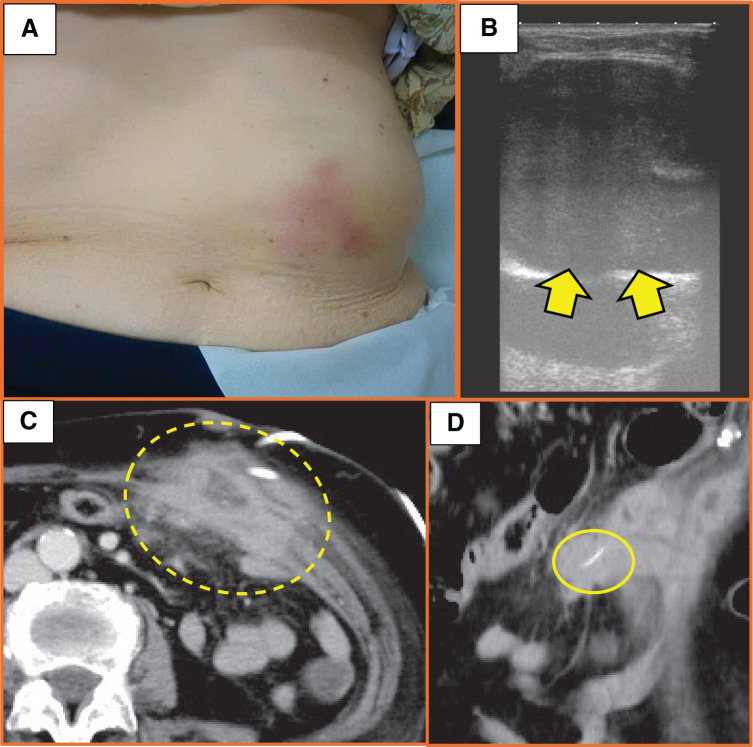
Physical findings and ultrasound 1 week after the first hospital visit, and enhanced CT images obtained on the 11th day after drainage. (A) The patient developed redness over the palpable mass. (B) Ultrasound shows increased fluid content in the lesion (yellow arrows). (C) Horizontal image shows that the low-density tumor shrank after abscess drainage (yellow dotted circle). (D) Sagittal image shows a new linear high-density structure (yellow circle).
